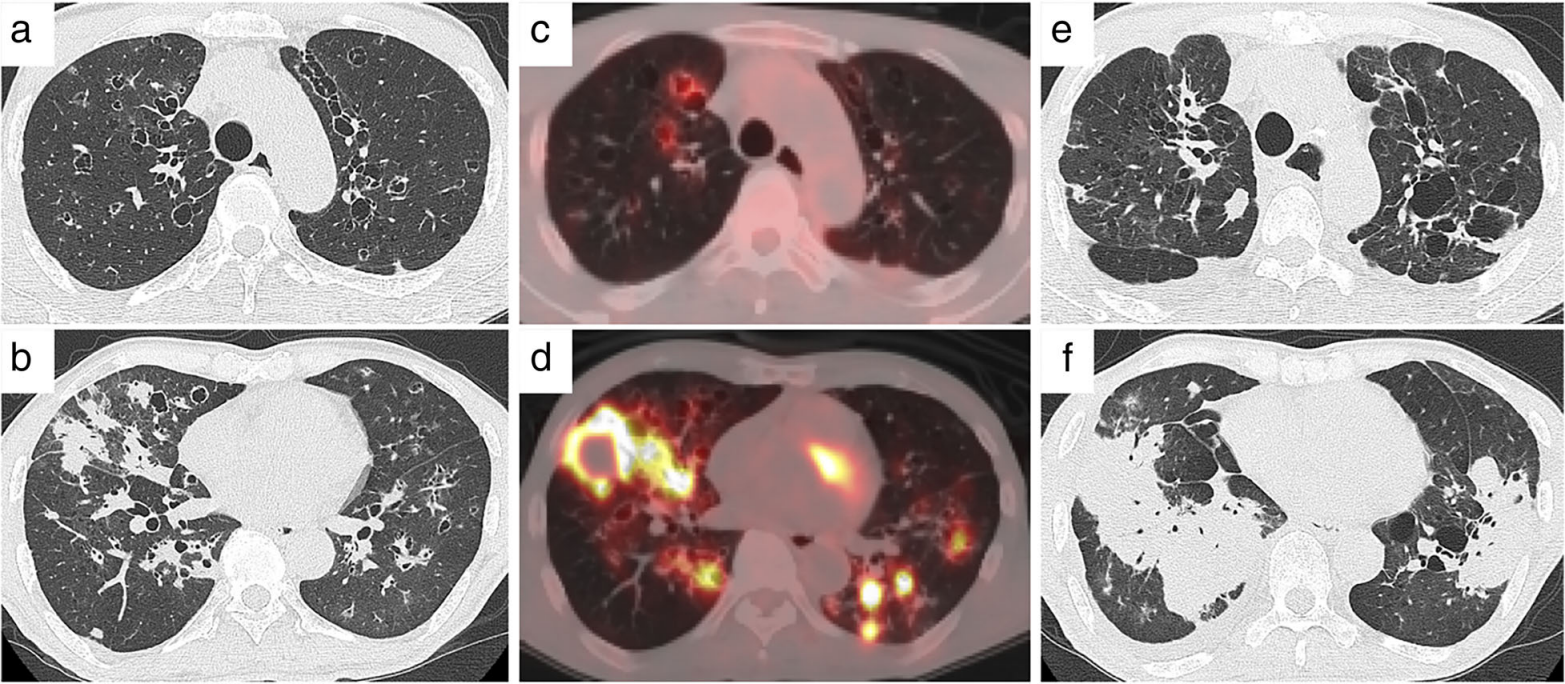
Chest radiological findings in a patient with silicosis. Chest computed tomography scans at the first visit show (a) cystic lesions and bronchiectasis at the upper lobes, and (b) multiple nodules at the right middle and bilateral lower lobes. Positron emission tomography shows high uptake of ^18^F‐fluorodeoxyglucose (c) into the cystic lesions and (d) the multiple nodules. Two years after the first visit, chest computed tomography scans show (e) the deterioration of cystic lesions and bronchiectasis at the upper lobes and (f) the emergence of several masses at the lower lobes
